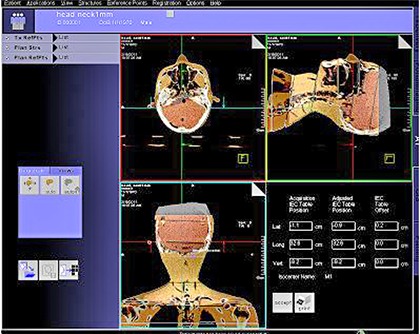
The MVision system automatically registered the CBCT images to the planning CT images and automatically performed shift measurement.
